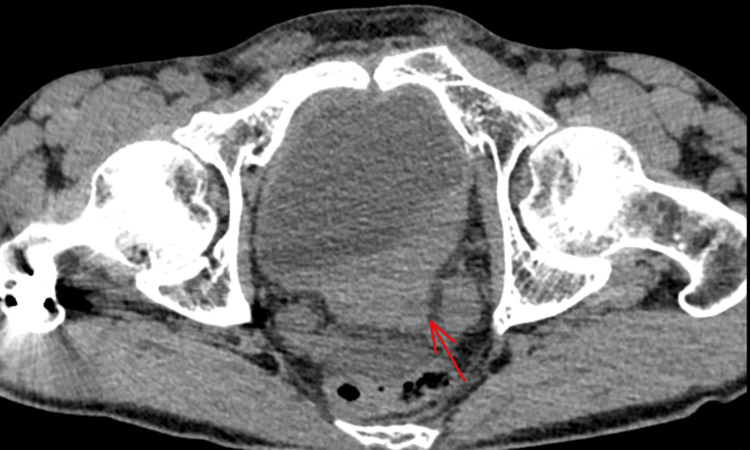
Axial section of a CT of the abdomen presenting a tumor of the left posterolateral wall of the bladder (red arrow).
